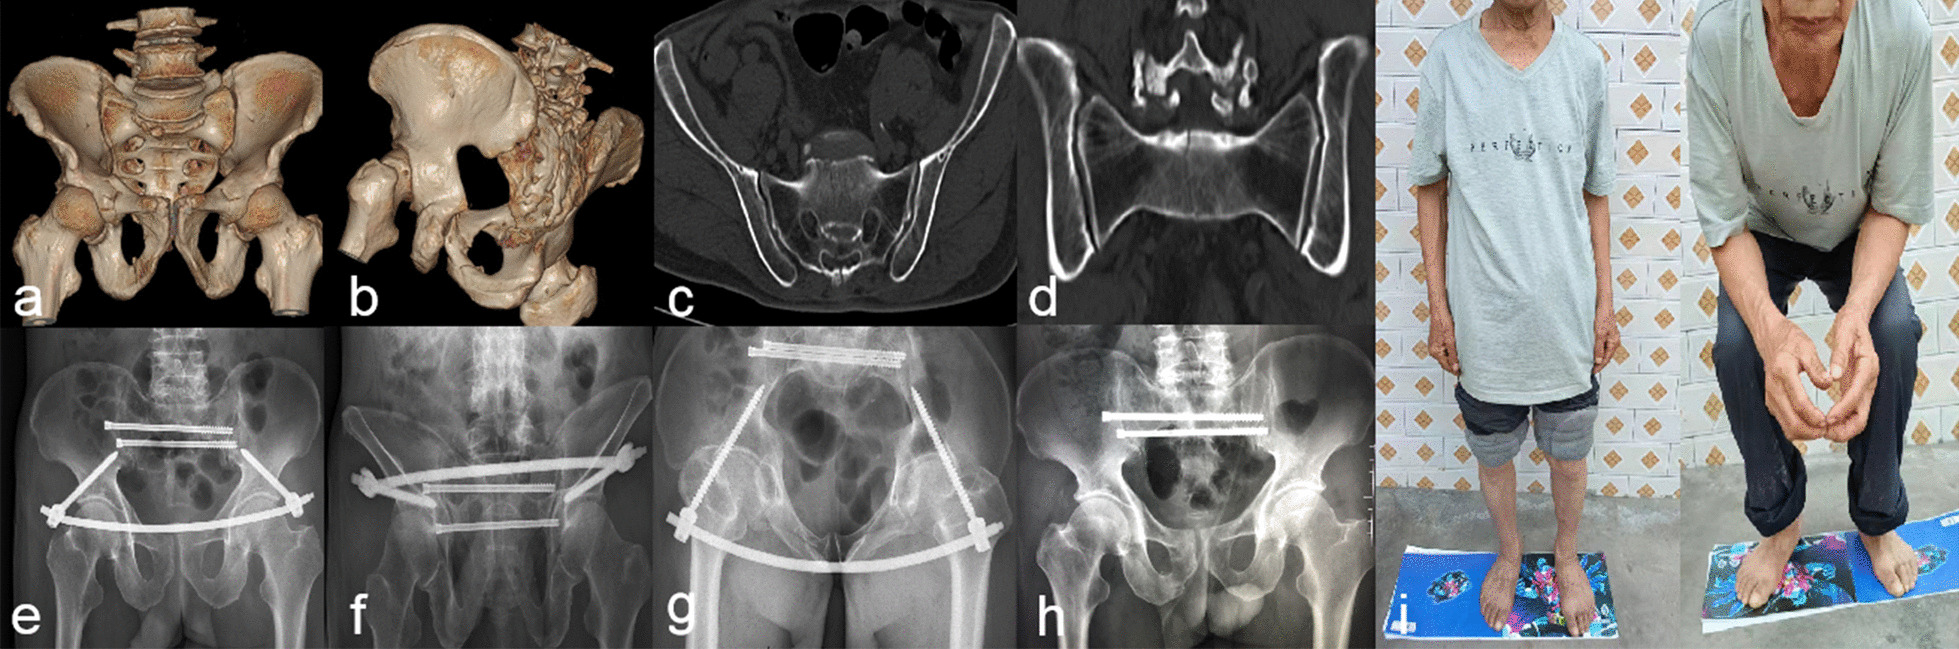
Typical case 2: A 82 years old male patient with pelvic ring fractures (AO/OTA type B) caused by a traffic accident. The patient had a combination of moderate osteoporosis, anaemia, hypoproteinemia, coronary heart disease and diabetes mellitus. Treatment patterns of hip fractures was used pre- and post-operatively. The patient was treated with a minimally invasive closed reduction and internal fixation by the INFIX 3 days after injury. a–d Preoperative X-ray film and CT 3D reconstruction showed comminuted fractures of the upper and lower branches of the pubis. e–g The pelvic AP, outlet and inlet X rays. h Post-operative radiograph at 5 months after the INFIX stent removal, showing good fracture healing. i Post-operative functional images at 12 months
